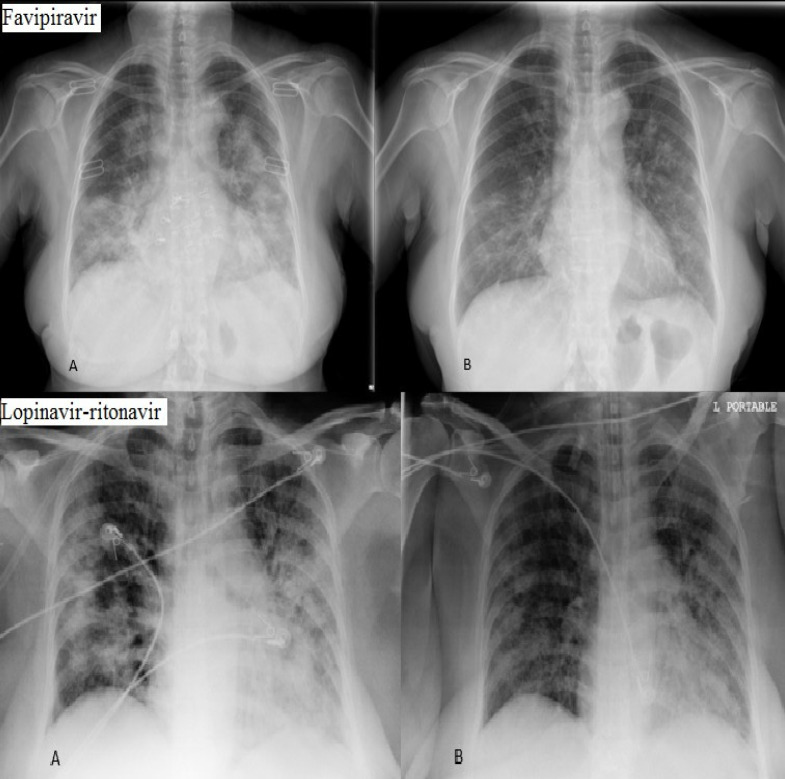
Chest X-ray improvement in two cases. (A: baseline, B: day 10)
